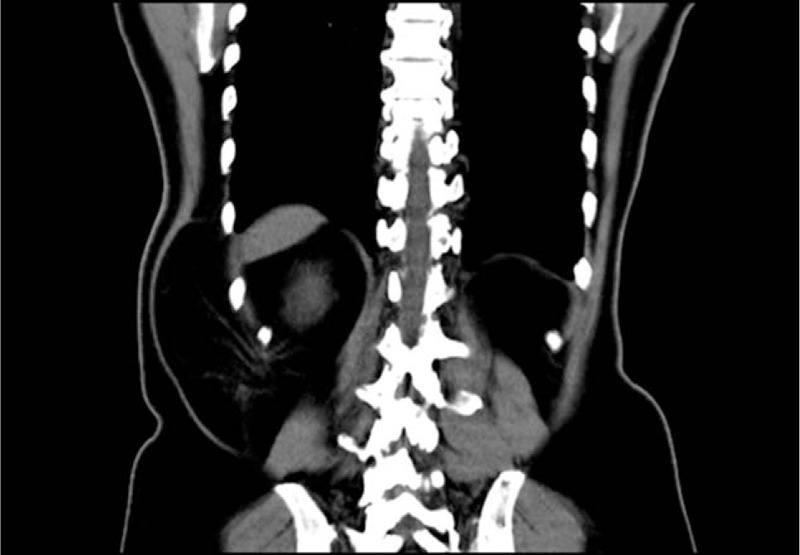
Coronal section of the CT scan showing the right lumbar hernia of the same patient as in Figure 1. CT = computed tomography.
